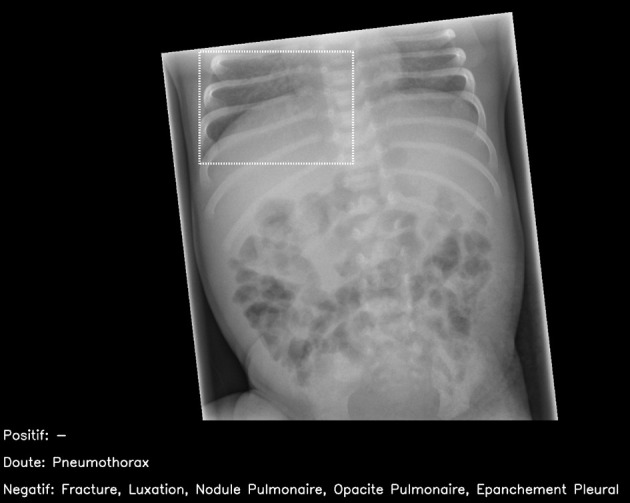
Normal paediatric abdominal radiograph interpreted by artificial intelligence (AI) candidate as having right basal pneumothorax with dashed bounding box (false positive result). This should have been identified as non-interpretable by AI. French translation: positif=positive; doute=doubt; epanchement pleural=pleural effusion; luxation=dislocation; negatif=negative; nodule pulmonaire=pulmonary nodule; opacite pulmonaire=pulmonary opacification
